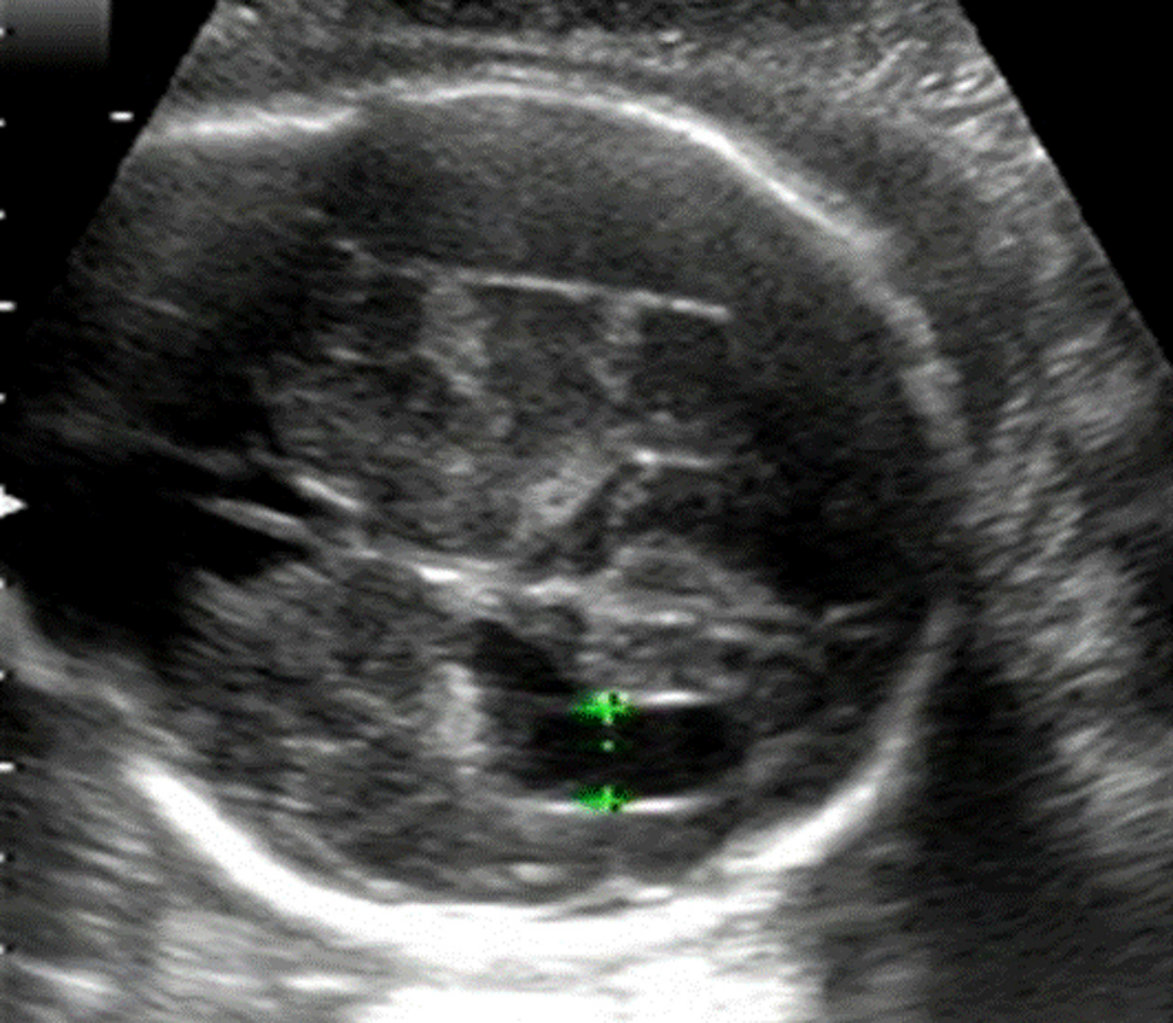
Head of the fetus at the 33 weeks of gestation. Transabdominal ultrasonography reveals enlargement of the left lateral ventricle and hematoma in the right subdural area.
